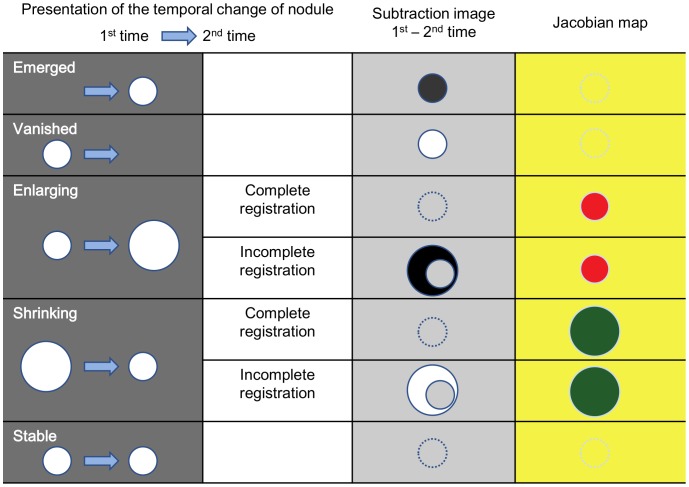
Temporal change in nodules and results of transformation in serial CT scans, with visual presentation in a subtraction image and a Jacobian map. The subtraction image shows gray for pixel value = 0, black for negative, and white for positive value. The color-coded Jacobian map shows green for Jacobian <1, red for Jacobian >1, and yellow for Jacobian = 1.
